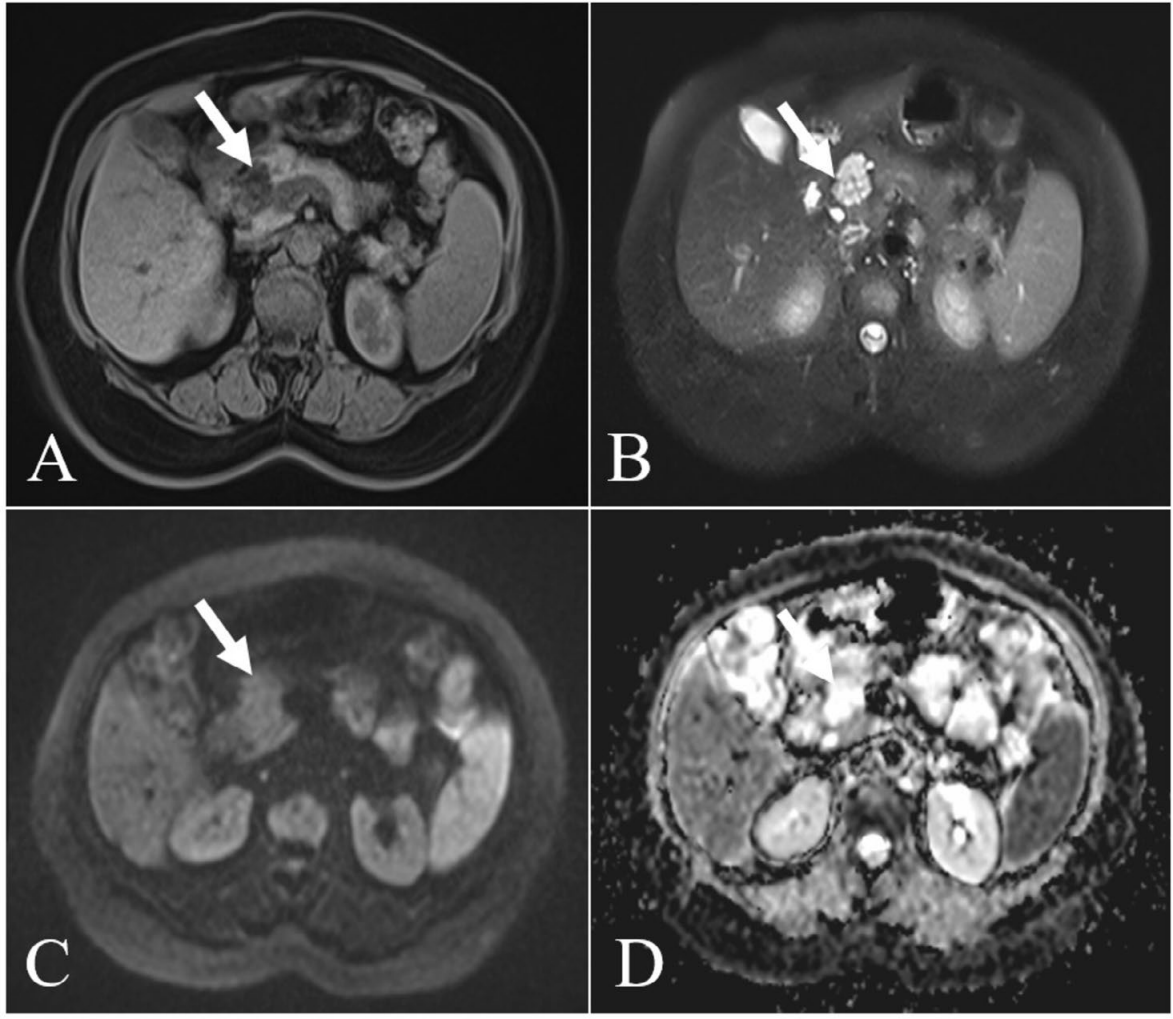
Magnetic resonance imaging (MRI) showed an abnormal signal nodule in the head of the pancreas, with isointensity and hypointensity on T1‐weighted images (A) and inhomogeneous hyperintensity on T2‐weighted images (B). The isointensity on diffusion weighted imaging (DWI; C) and high apparent diffusion coefficient (ADC; D) values indicated a low impedance to diffusion of water molecules within the lesion. In addition, the lesion was not connected to the main pancreatic duct and there was no dilatation of the main pancreatic duct.
